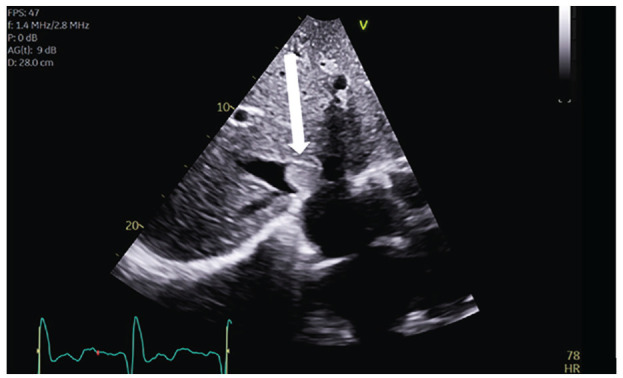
Transthoracic echocardiogram subcostal view revealed severe left ventricular dilation with an ejection fraction of 25%–30% and a mobile echodensity of 1.7 cm × 2.4 cm (arrow) at the junction of the inferior vena cava and the right atrium.
